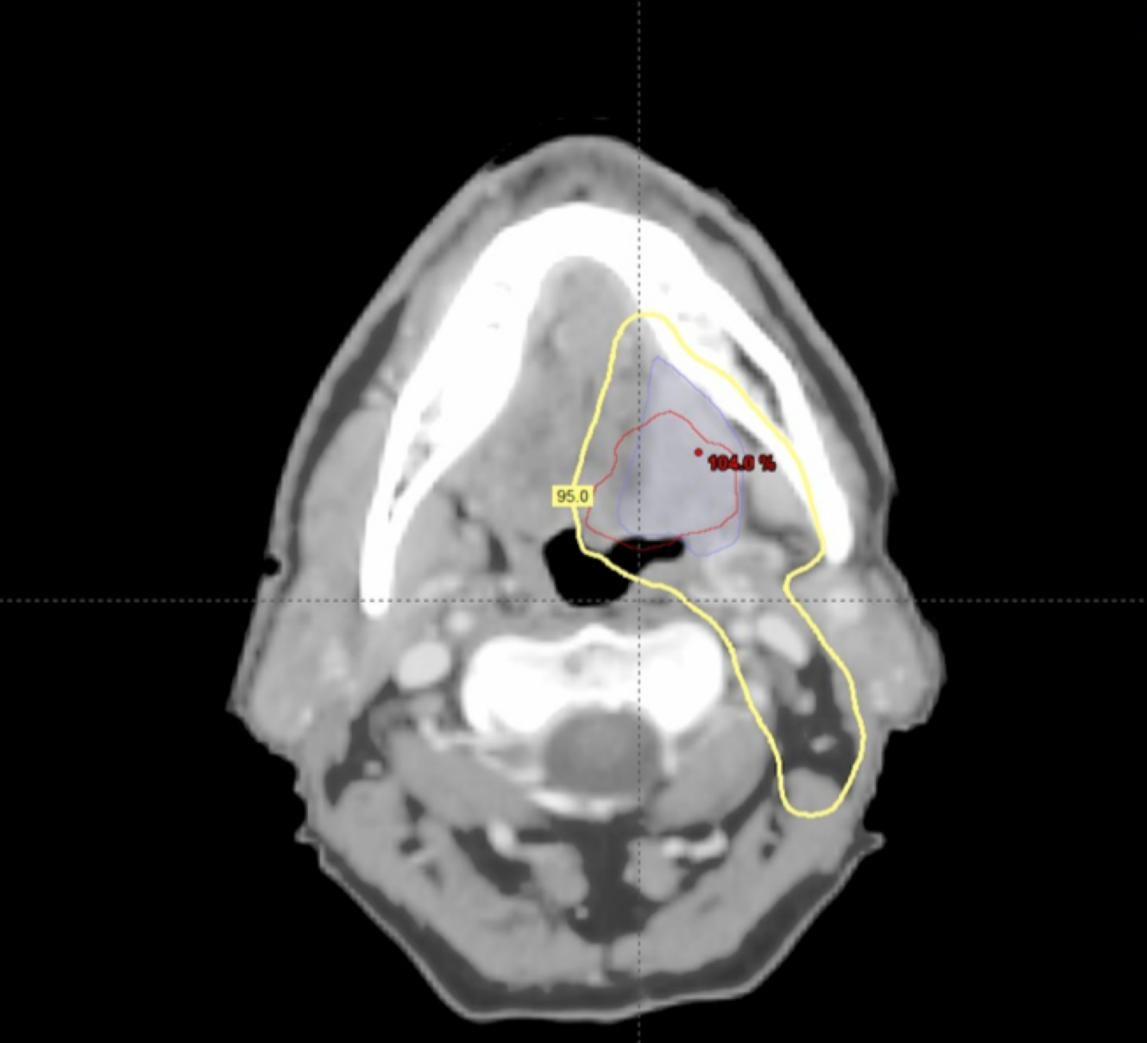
In-field recurrence T2 N1 carcinoma of the left tonsil treated with concurrent chemoradiotherapy; Original gross tumor volume (blue line); Delineation of recurrence (red line) after co-registration with original planning computed tomography; 95% isodose line (yellow).
